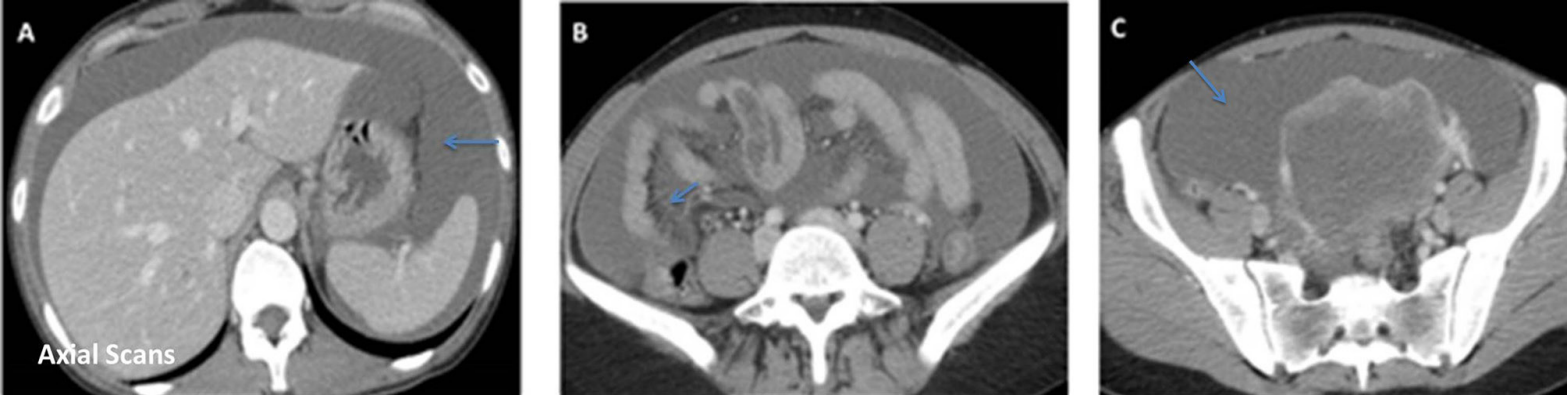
Axial scans of emergency abdominal CT, which shows abundant ascitic liquid
